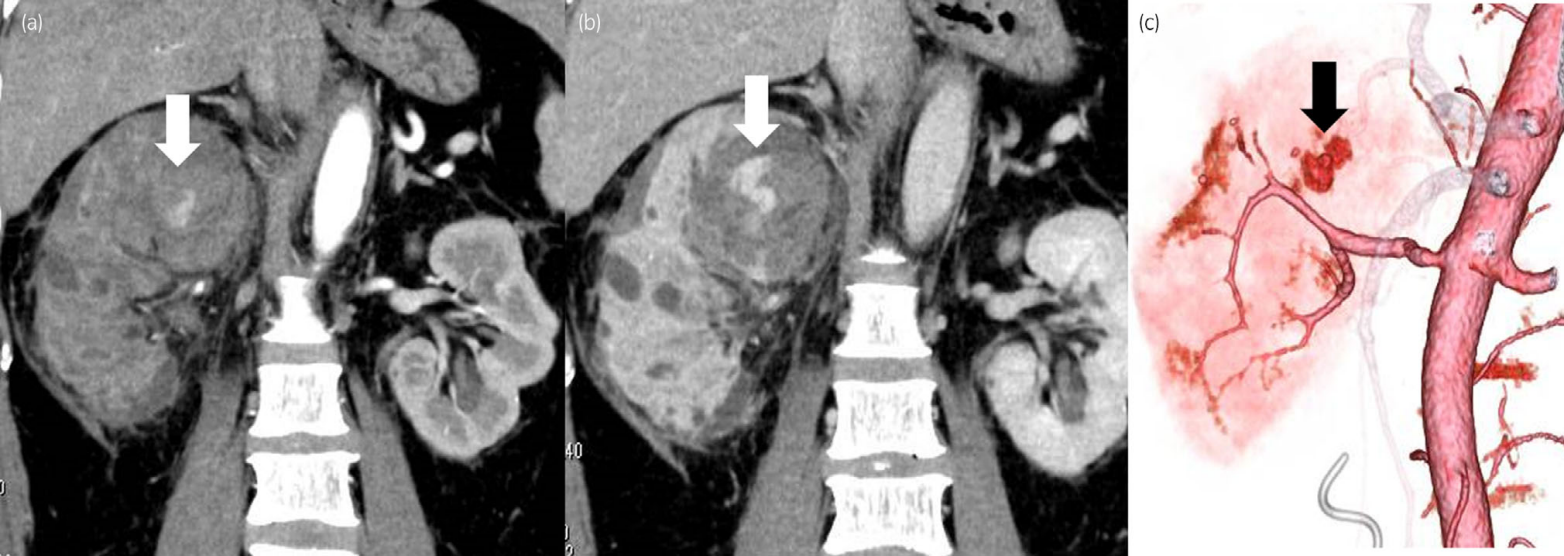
Contrast‐enhanced Computed Tomography on day 9 demonstrates a ruptured pseudoaneurysm. (a) Arterial phase; (b) Venous phase; (c) 3D‐Compued Tomography.
